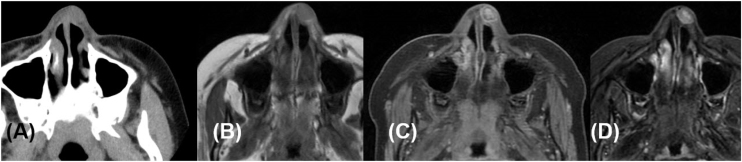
CT scan (A), T1 (B), contrast-enhanced T1 (C), and T2 (D) MRI show well demarcated ovoid benign mass lesion, measuring 14 × 12 mm in size at the left nasal dorsum with inhomogeneous hyperintensity in T2 image.
